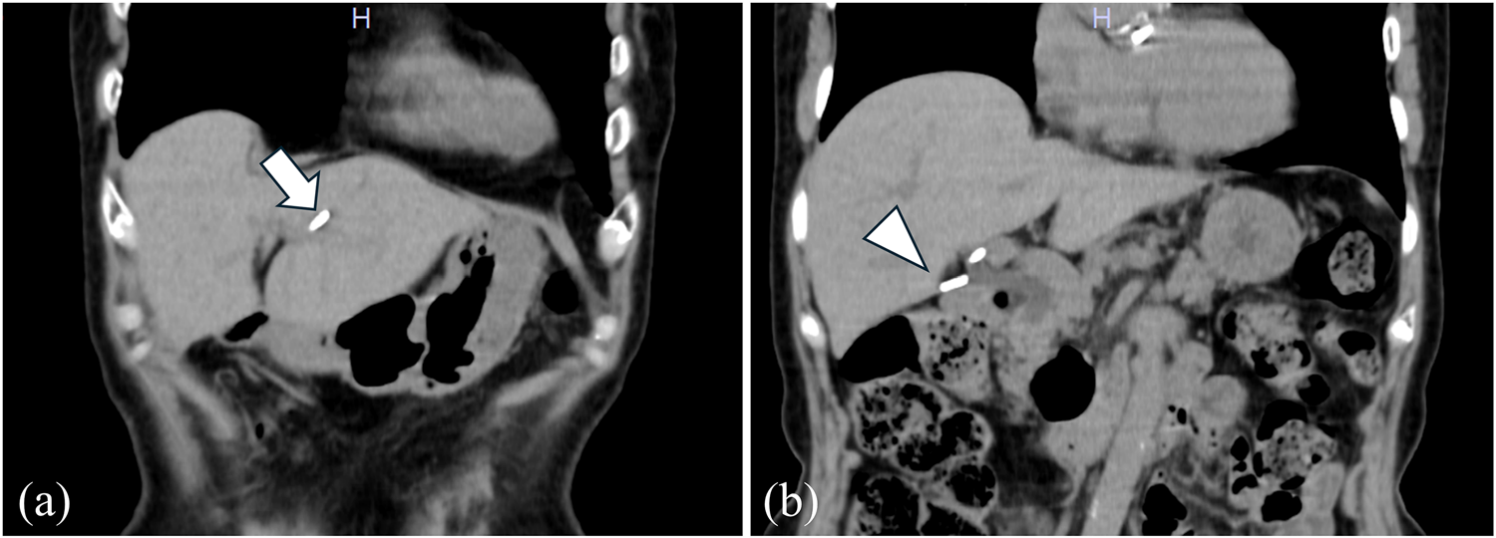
Non‐contrast abdominal computed tomography (CT) scan 1 year after cholecystectomy (coronal view). (a) One of the two clips is misplaced in the left hepatic duct, and the other is misplaced in the common hepatic duct (arrow). (b) Another clip remains in the cystic duct (arrowhead). Figures 1 (a) and (b) are both 497 × 415 mm, 330 dpi.
